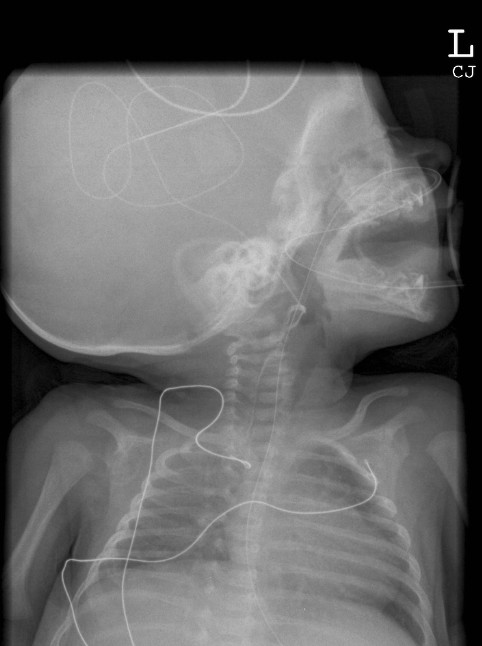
Radiograph post peripherally inserted central venous line insertion.
